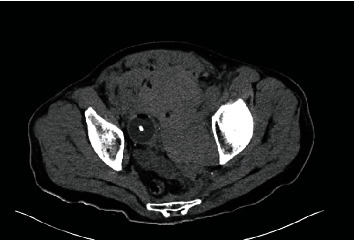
CT pelvis with cystogram at our hospital (axial): displaced bladder; poor cystogram without extravasation.
